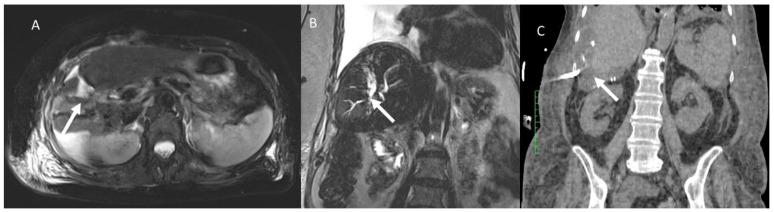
Woman 58 years at 1-week follow-up after microwave ablation of liver metastasis. MRI assessment. (A) Sampling perfection with application optimized contrasts using different flip angle evolution (SPACE) T2-weighted fat sat sequence in axial plane and (B) SPACE T2-weighted fat sat sequence in coronal plane, arrow shows biloma. In (C) CT evaluation (multi-planar reconstruction coronal plane) of biloma drainage (arrow).
